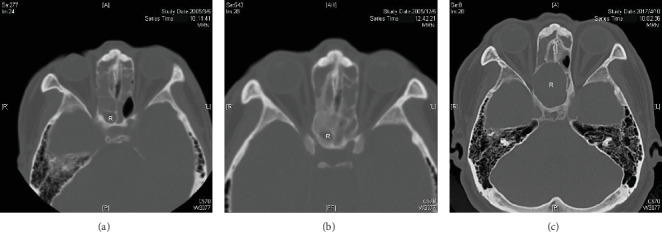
Progression of the right diseased sphenoethmoidal cell of the patient who developed visual loss in similar cuts of CT images in Aug. 2005 (a), Dec. 2005 (b), and Apr. 2017 (c). R: right sphenoethmoidal cell.
